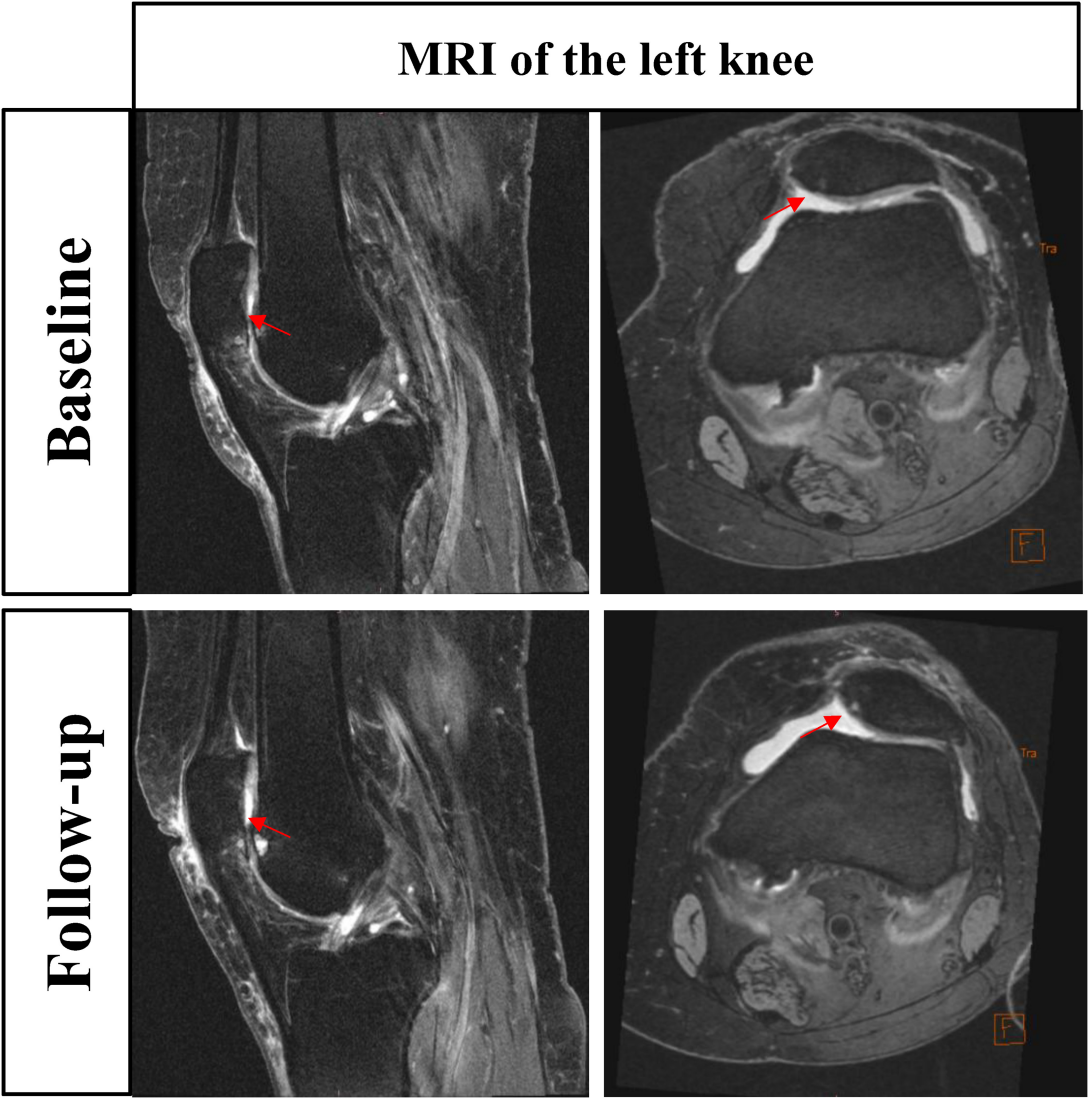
MRI images depicting patellofemoral cartilage changes in the knee of a 72-year-old participant engaged in middle-volume stair climbing after 2 years.
